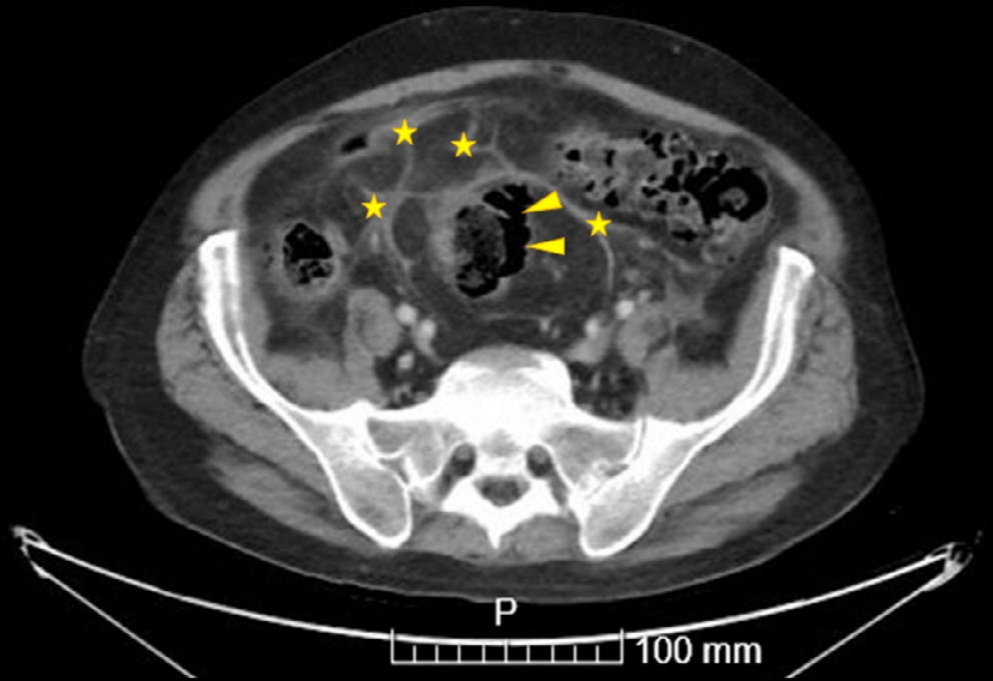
Abdominal CT scan performed during cycle 4. The yellow arrows indicate the discontinuity in the colonic wall at the sigmoid level. The yellow stars highlight the fat stranding suggestive of surrounding peritonitis.
